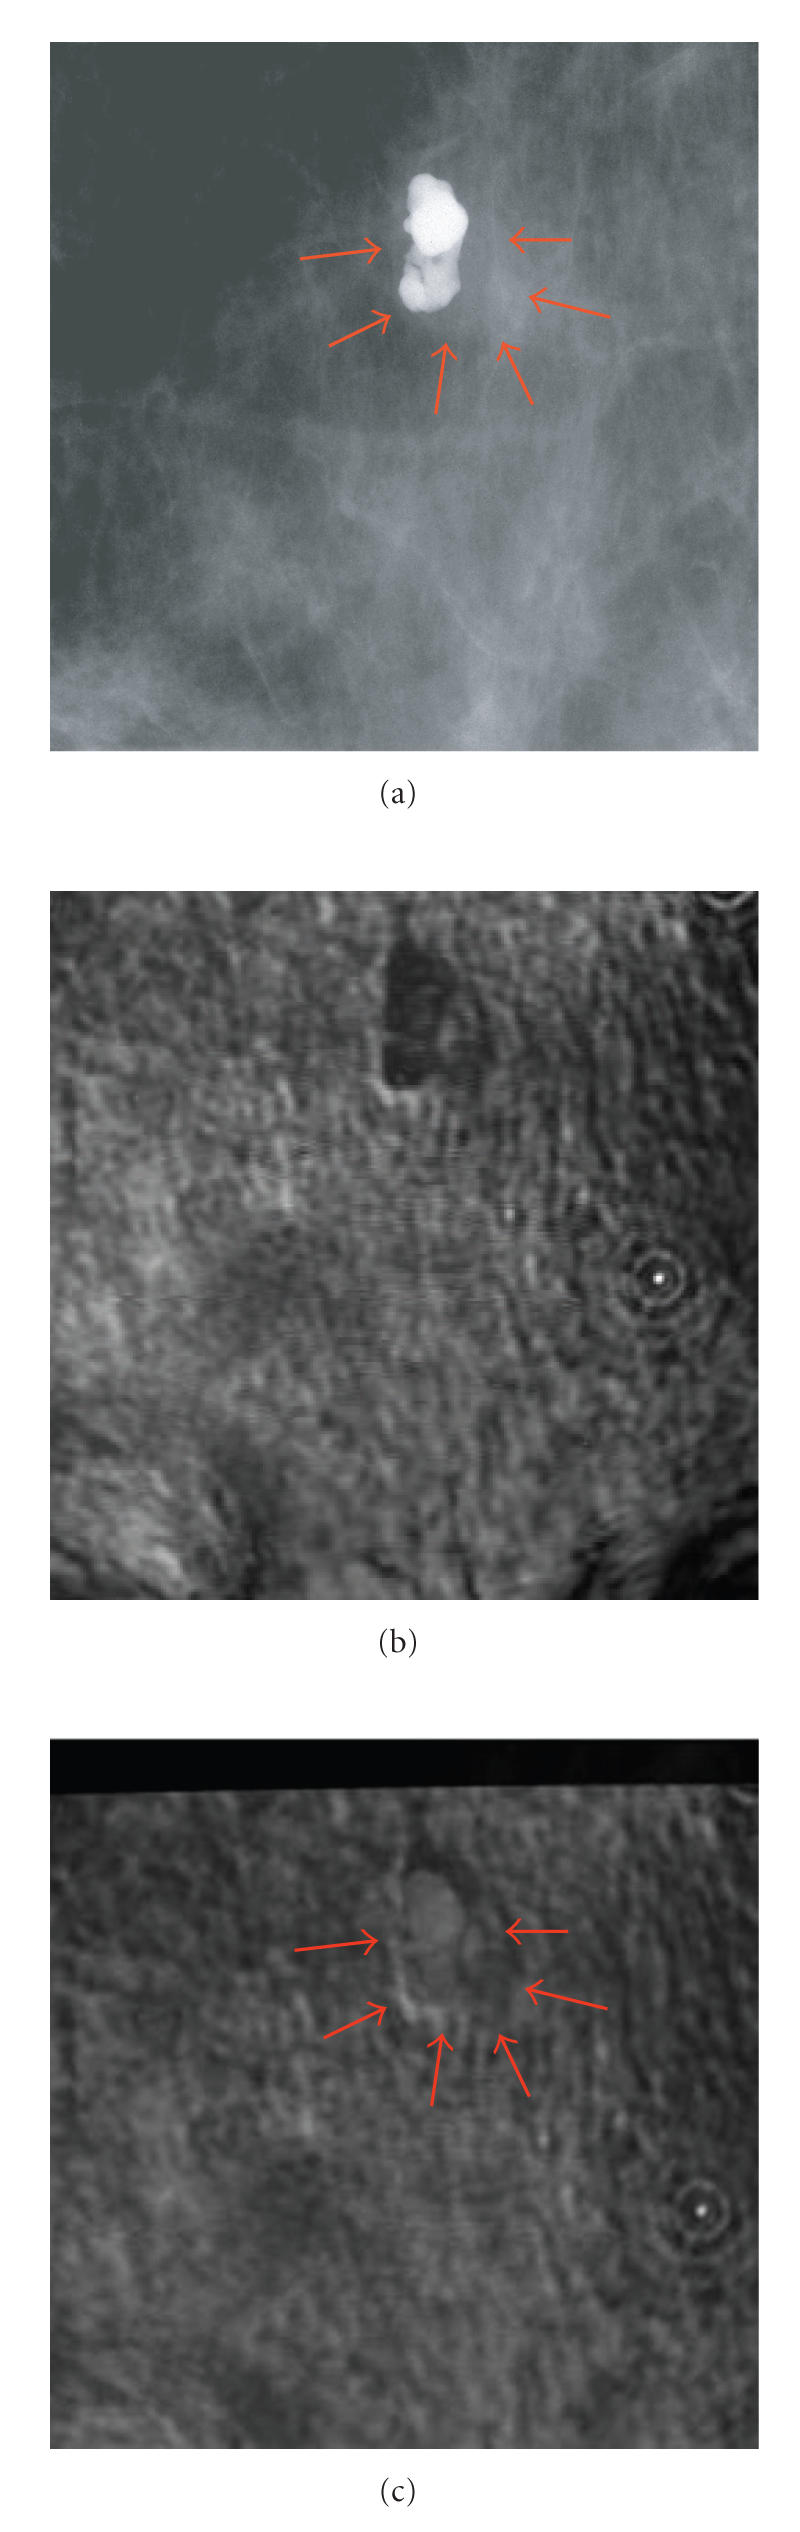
The breast image with fibroadenoma: (a) marked X-ray by
